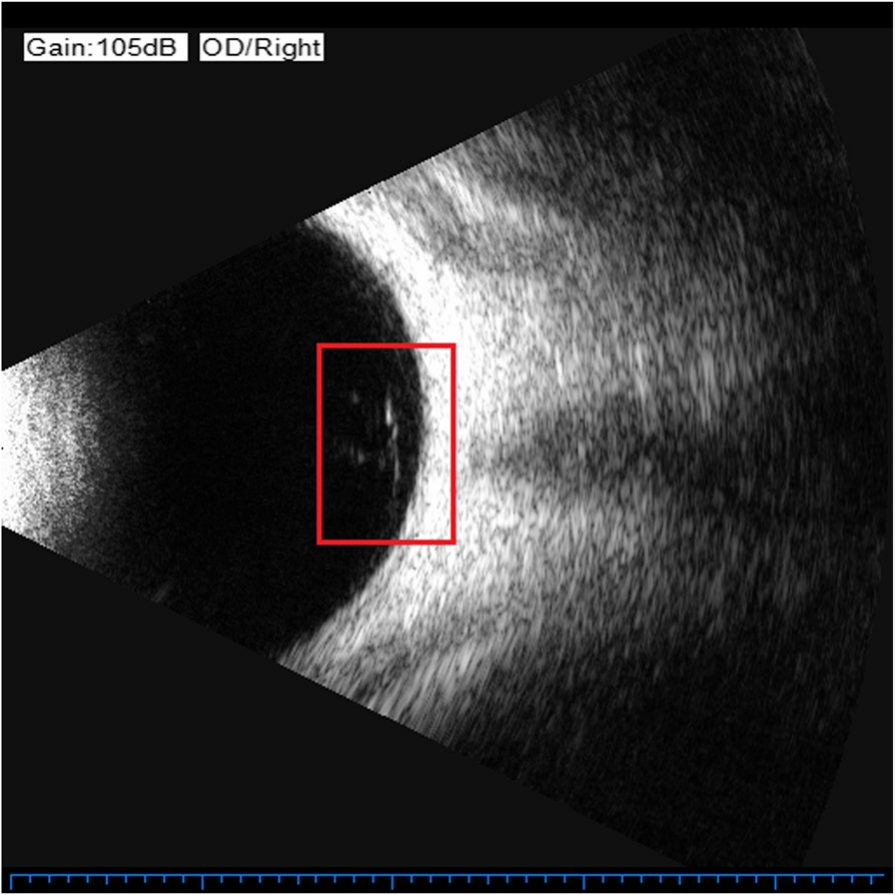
VO fundus ultrasound image
